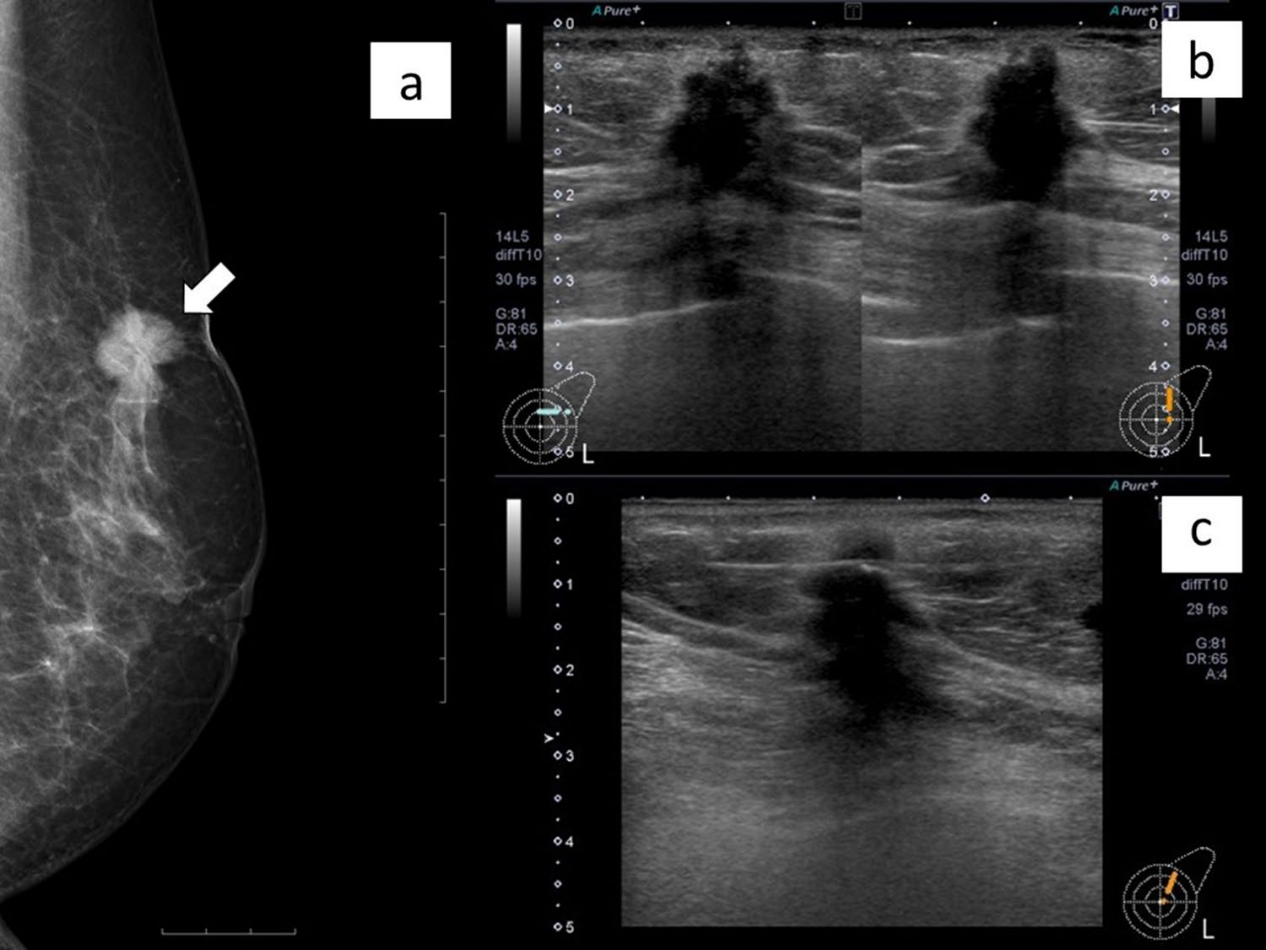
Mass (invasive ductal carcinoma) diagnosed using core needle biopsy. A woman in her 70s who was aware of a breast mass. Mammogram showing a spiculated mass with skin indentation in the right breast (arrow) (a). Ultrasonogram showing an irregularly shaped, indistinct mass 16 mm in diameter that was diagnosed as a BI-RADS category 5 lesion (b). A 16-gauge spring-loaded core needle biopsy was performed, and the lesion was diagnosed as invasive ductal carcinoma of the breast (c)
